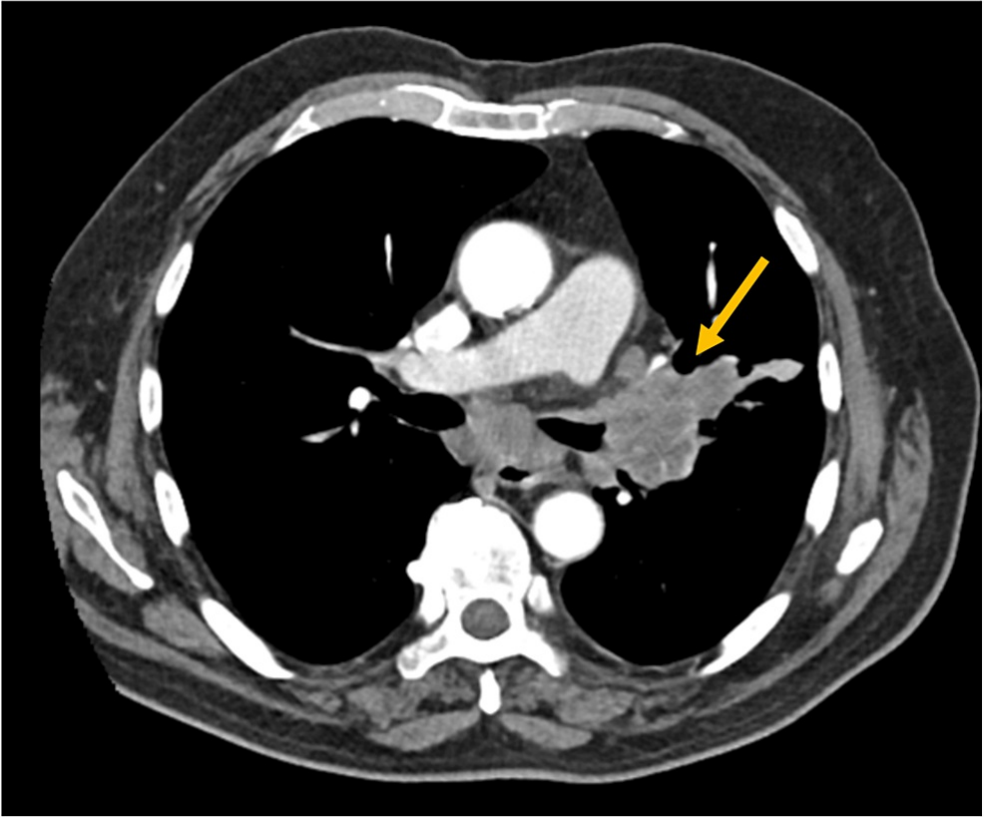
Thoracic CT scan showing a pulmonary mass invading the left pulmonary artery and vein
